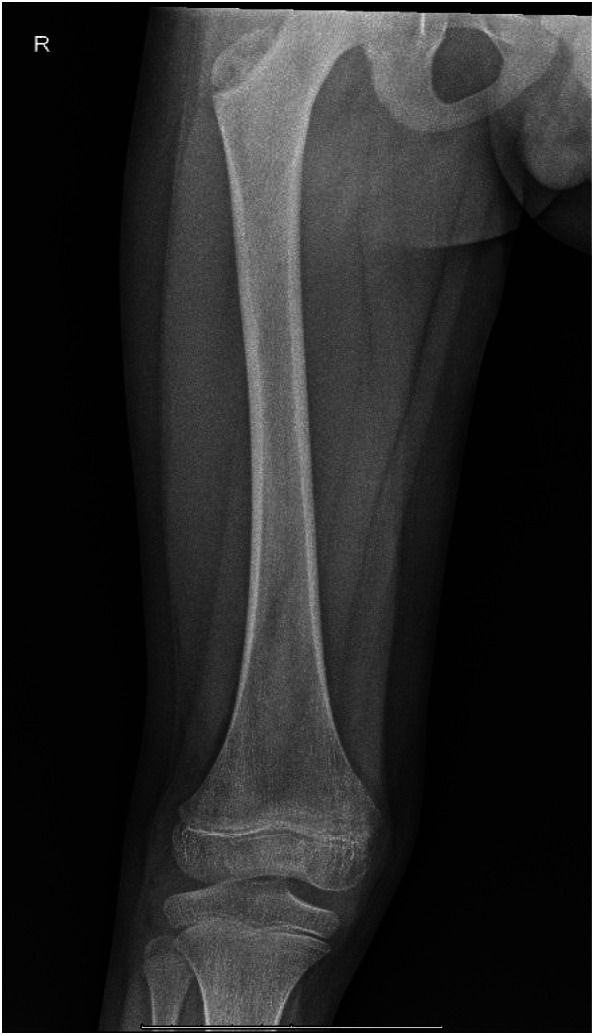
Radiograph of the right femur upon emergency department presentation with no acute abnormalities.
